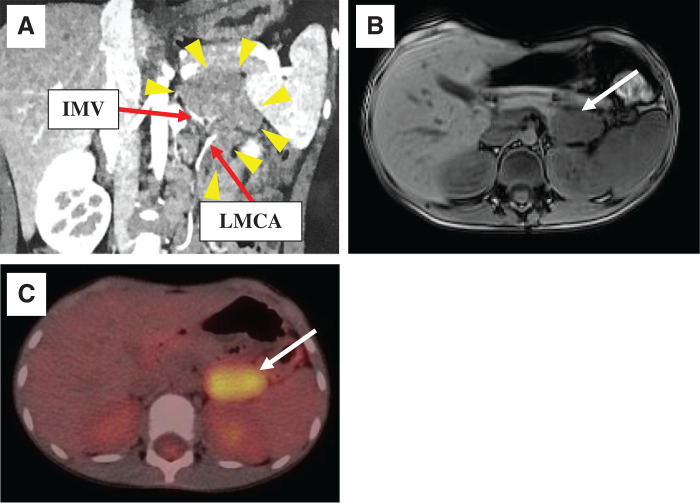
Imaging studies before operation. (A) Abdominal enhanced CT showed a 45 × 25-mm clear-boundary, smooth surface, and weakly enhanced tumor (arrowheads). The tumor was adjacent to the IMV and the LMCA without encasement. (B) Abdominal MRI showed a 38-mm clear-boundary mass between the distal pancreas and the left kidney, which exhibited uniformly low intensity on T1-weighted imaging (white arrow). (C) FDG-PET. An abnormal accumulation of FDG was detected between the distal pancreas and the left kidney (white arrow). FDG-PET, fluorodeoxyglucose PET; IMV, inferior mesenteric vein; LMCA, left branch of the middle colic artery
